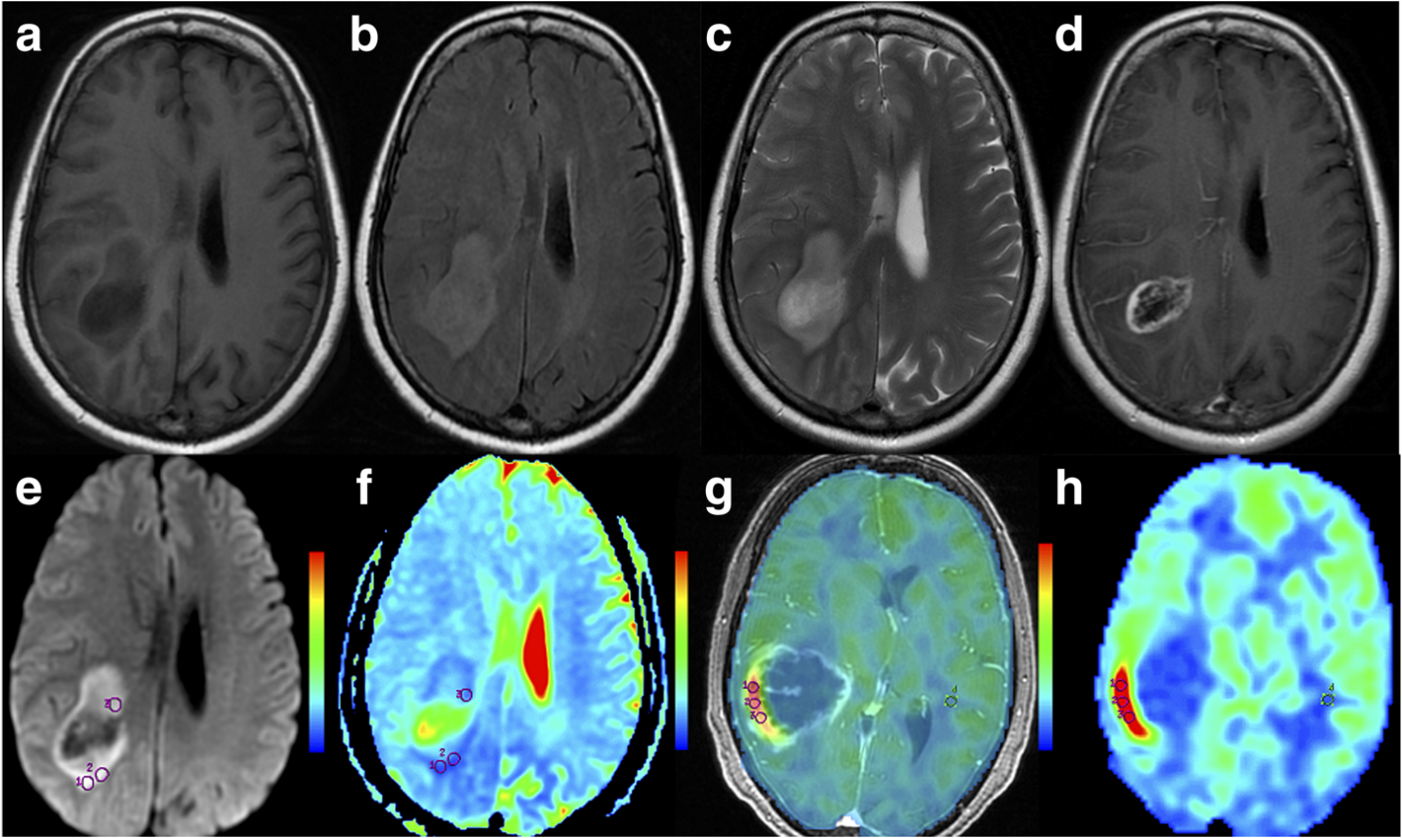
Structural (a, b, c and d) and advanced (e, f, g and h) MR images obtained from a 55-year-old male showing right temporal and parietal lobe GBM without MGMT promoter methylation. a Precontrast T1-weighted image. b Fluid-attenuated inversion recovery image. c T2-weighted image. d Postcontrast T1-weighted image. e DWI map. f ADC map: the mean ADC value of the three small ROI was 0.661 × 10^− 3^ mm^2^/s. g The map fused CBF and 3D–T1WI/C. h CBF map: the mean rCBF of the three small ROI was 10.64
